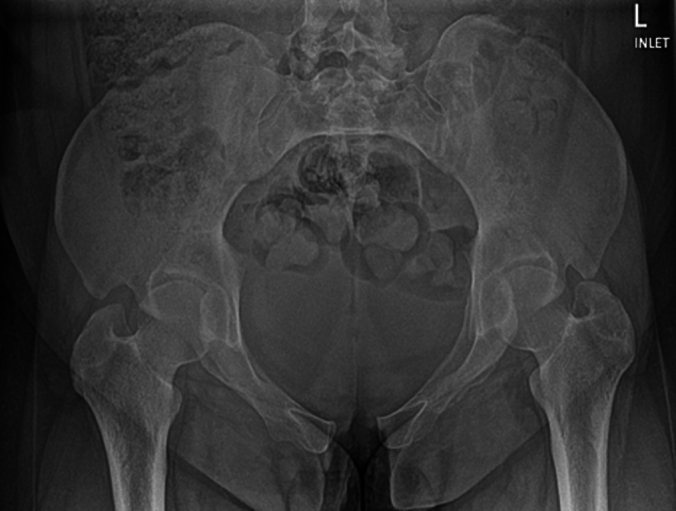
x-ray; an AP inlet view of the pelvic.
